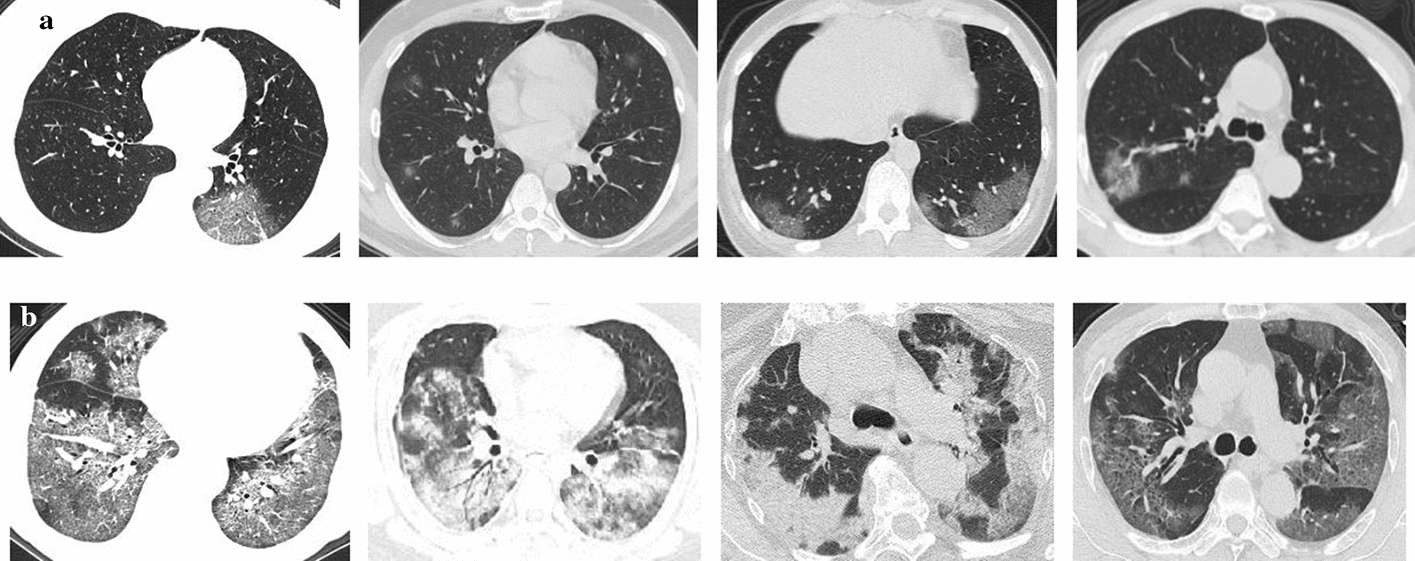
Sample CT scans of COVID-19-infected patients: a non-severe cases; b severe cases
